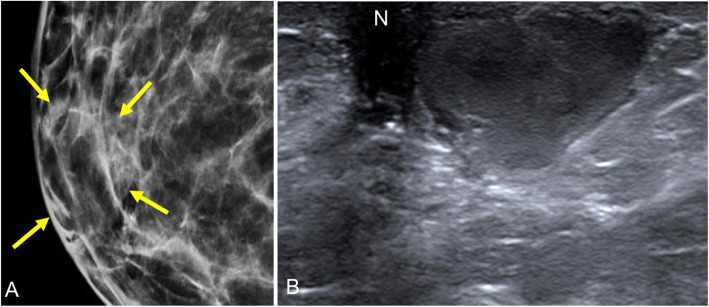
Epidermal inclusion cyst. A 37-year-old woman with a palpable retroareolar nodule in her right breast, without inflammation. a Synthesized 2D mammogram (craniocaudal view) shows a well-defined isodense nodule measuring 25 mm (arrows) adjacent to the nipple. b Ultrasound shows a well-defined hypoechogenic nodule below the skin of the right areola (*N* nipple)
